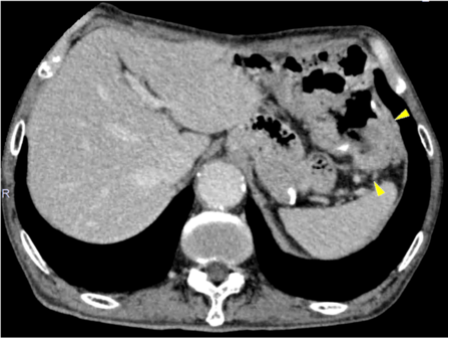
Contrast-enhanced computed tomography (CECT) showed a splenic flexure colon tumor at the staple line (arrows).
